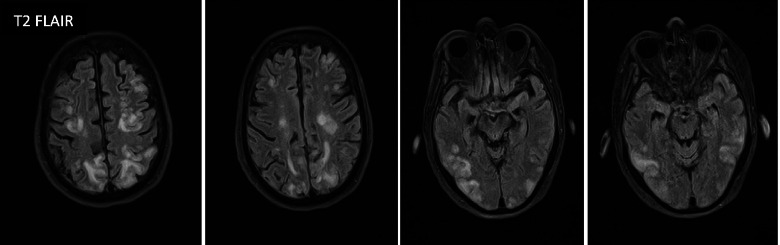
Axial T2-weighted, FLAIR images, showing extensive patchy and confluent T2/FLAIR hyperintensity of the subcortical U-fibers, most concentrated in the occipital and parietal lobes, but also visualized in the frontal lobes, bilaterally on day of admission
